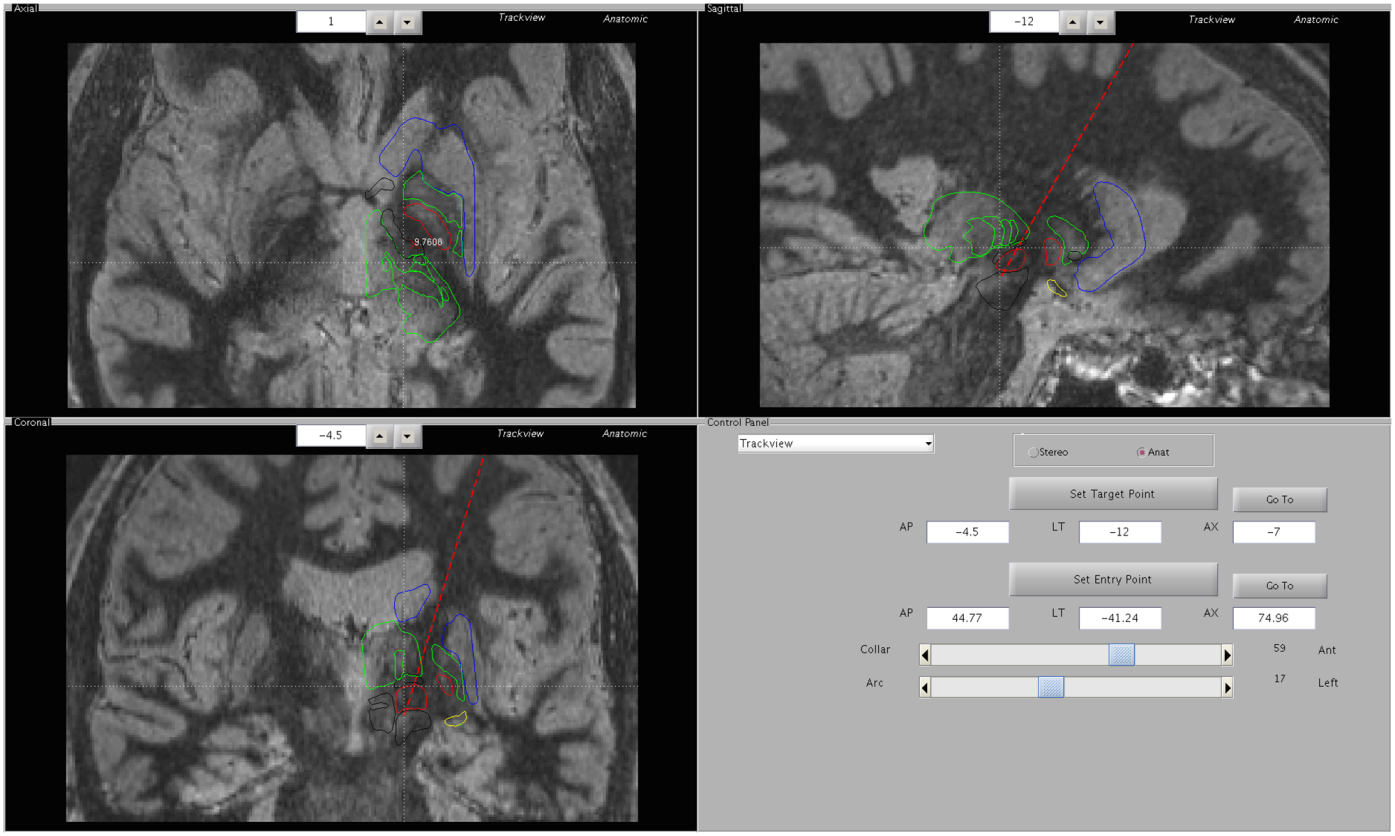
Graphical depiction of the measured neuroanatomical position of a DBS lead. The precise position of the implanted DBS lead is measured on a high-resolution CT scan acquired a few weeks after implantation and fused to the pre-operative targeting images (FGATIR MRI sequence with patient-specific deformed 3D atlas overlay).
